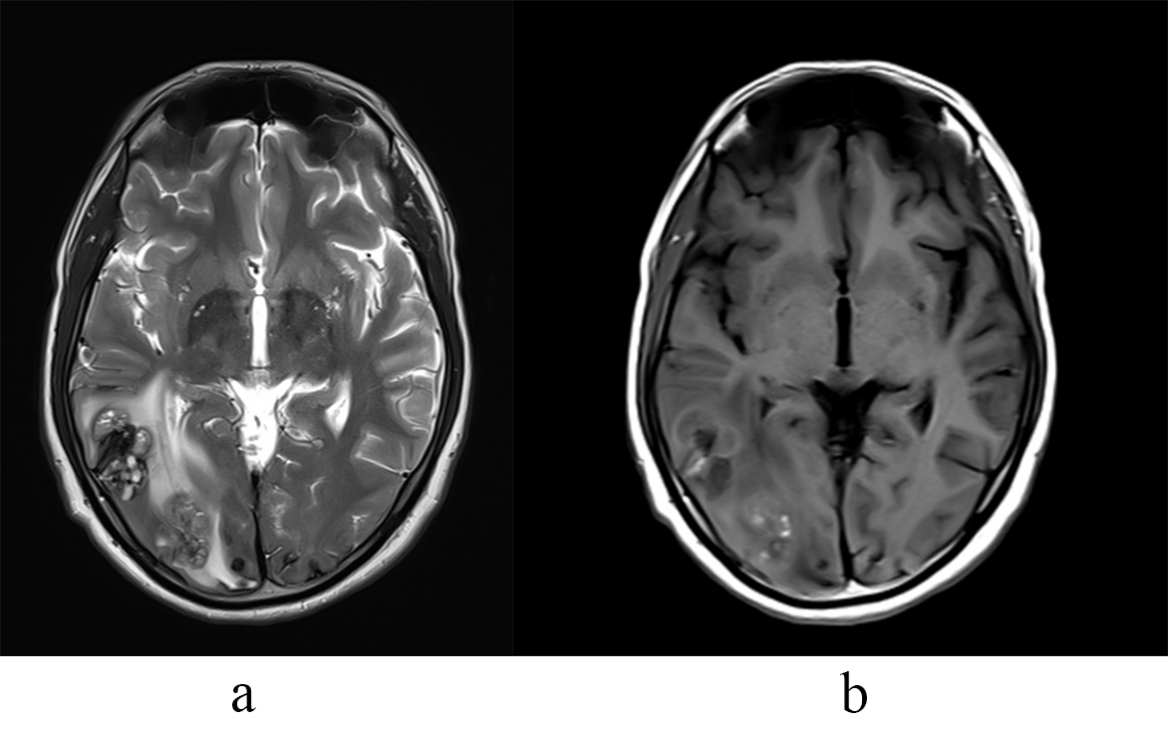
Right temporo-occipital lesions on MRI. (a) T2W MRI shows right
